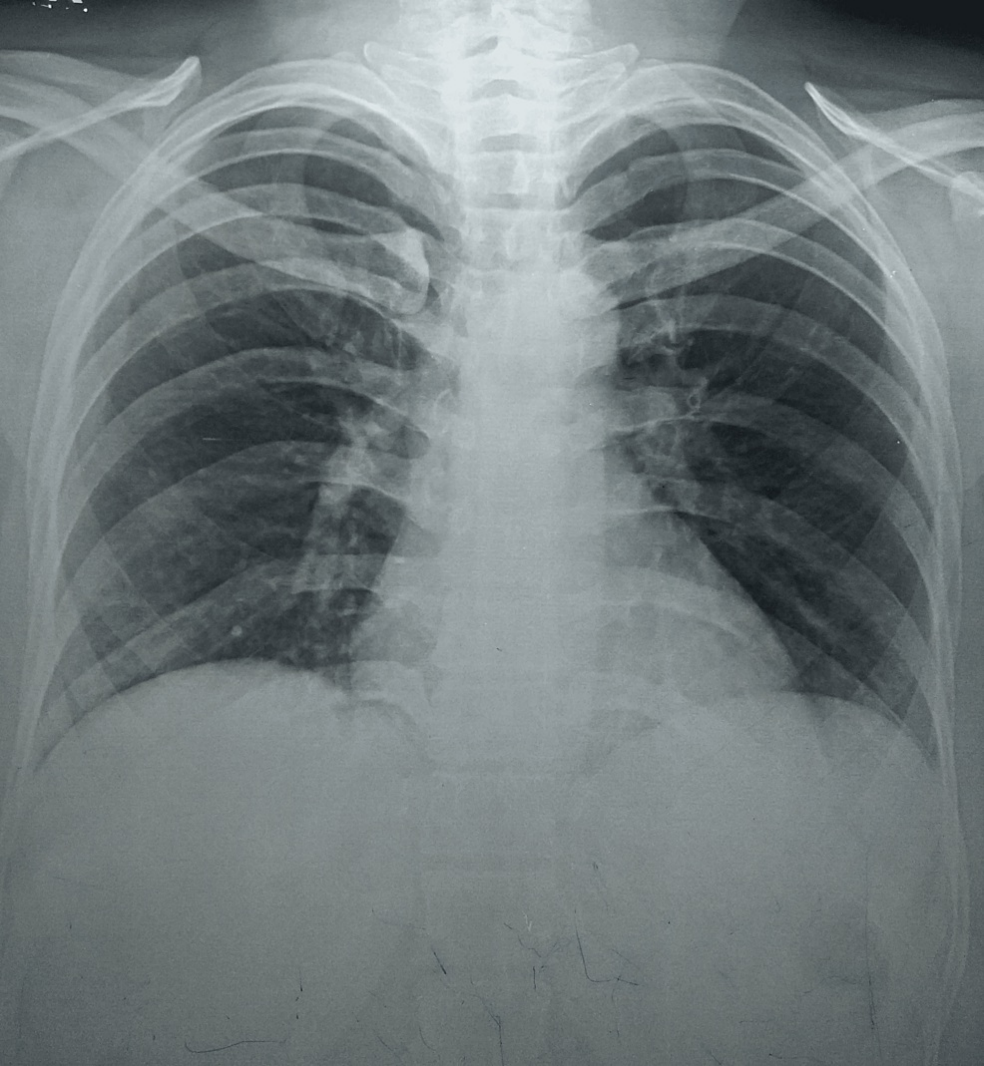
A normal chest radiograph posteroanterior (PA) view
